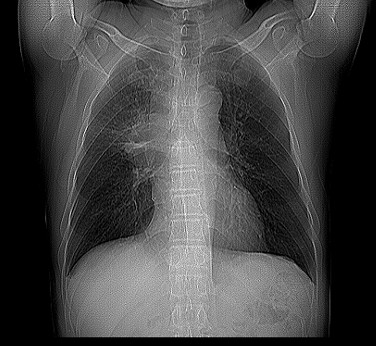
A high density shadow in the right hilar (X-ray).
